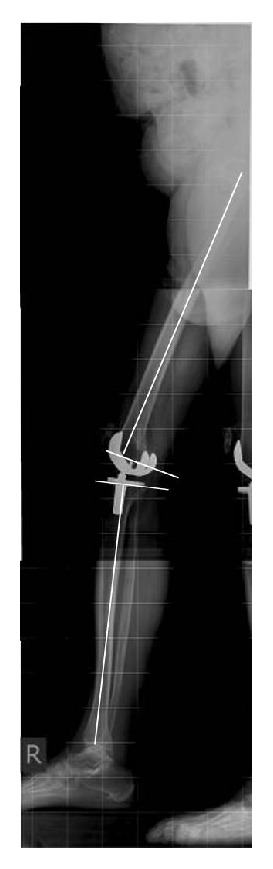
Long leg standing X-rays were performed in lateral view. The femoral flexion/extension angle and the tibial slope of the prosthesis were determined.
